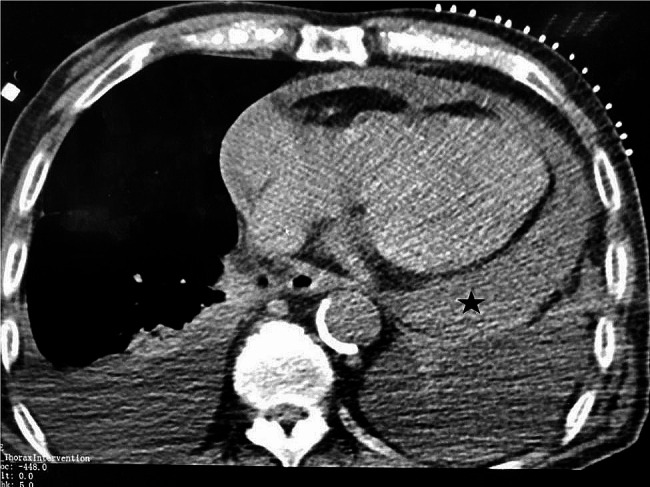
Postoperative chest CT examination. Chest CT examination revealed the presence of pericardial effusion (star)
